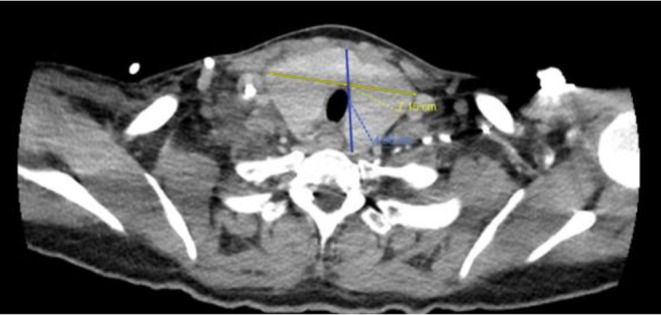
CT chest with contrast showing enlarged thyroid gland approximately 7 × 5 cm in size.
